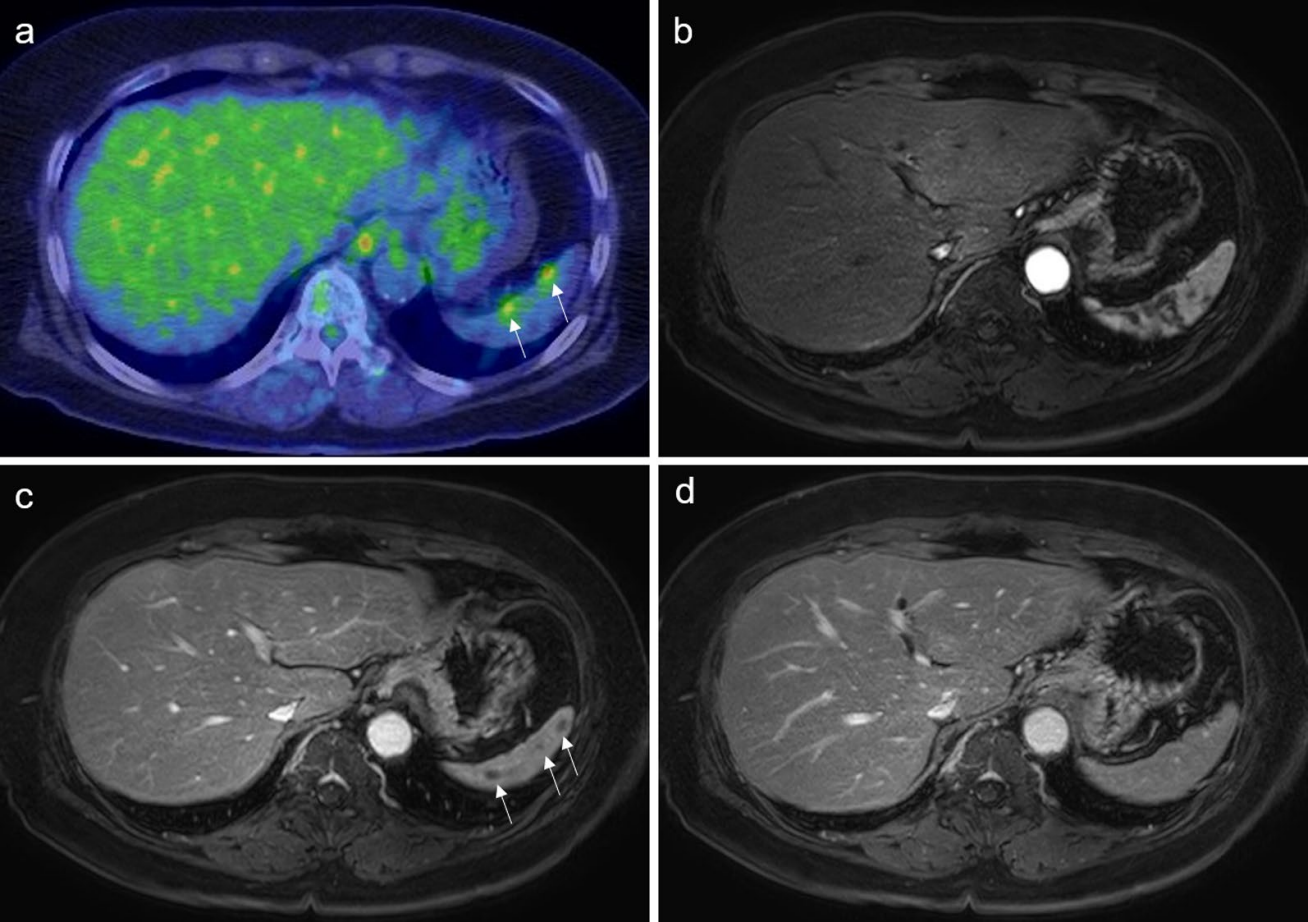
PET/CT and MRI findings of case 1. a PET/CT shows little 18F-fluorodeoxyglucose uptake at the site of the mass (maximum standardized uptake value: 4.11–4.95) in case 1. b MRI shows speckled contrast within the spleen during the early phase. c MRI during the late phase shows several ring-enhanced nodules in the spleen. d MRI shows no obvious nodules during the delayed phase. *PET/CT* positron emission tomography/computed tomography, *MRI* magnetic resonance imaging
